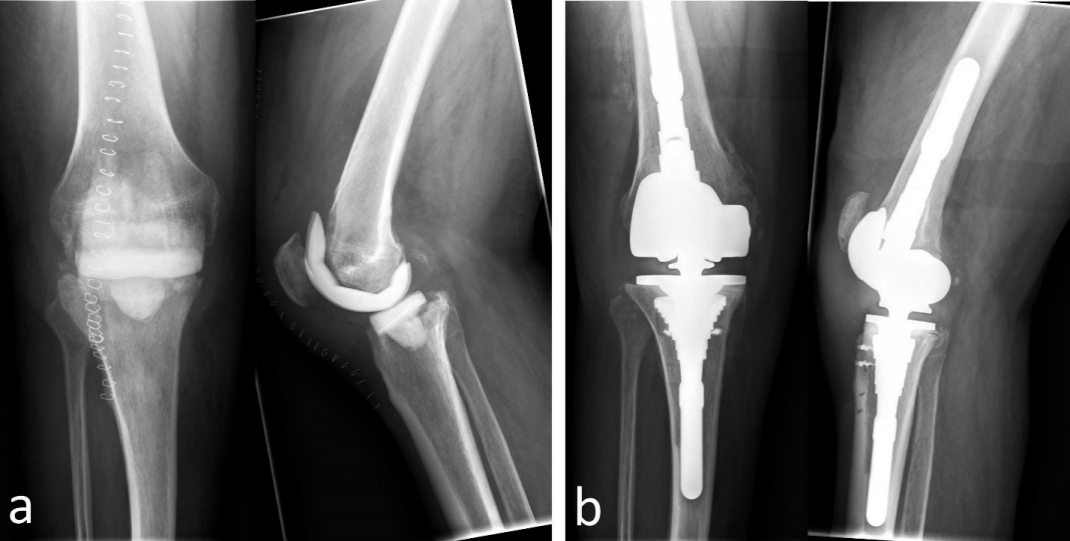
62-year-old patient with TKR infection right. a) Radiograph of the knee anterior-posterior and lateral after implantation of a movable spacer (knee spacer AGC Style Company BiometOrthopedics Inc. Warsaw, U.S.A.). b) X-ray of knee anterior-posterior and lateral after re-implantation of revision-TKR (Typ LCS revision, Company DePuy Synthes, West Chester, PA, U.S.A.)
